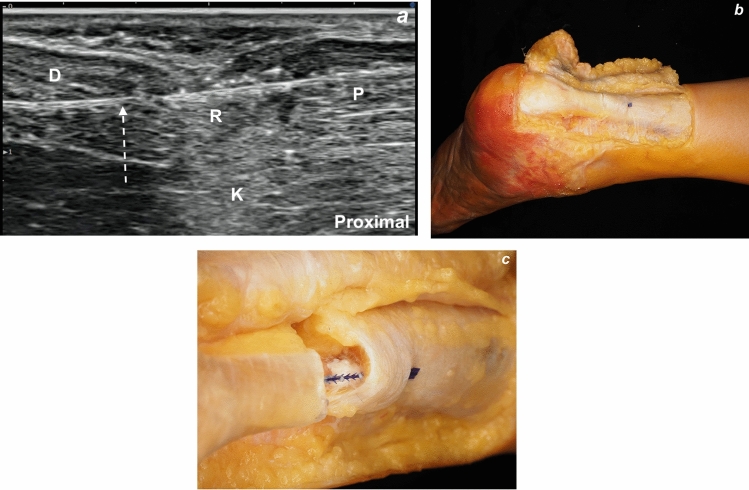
a, b, c Confirmation of tendon ends approximation following ultrasound-guided repair. a Ultrasound image of the Achilles tendon in the longitudinal axis, obtained prior to dissection, showing appropriate approximation of the tendon ends without residual gapping. b Cadaveric image of the dissected Achilles tendon in a resting position, showing that the tendon ends were in direct contact, confirming successful reapproximation. c Intratendinous dissection showing that the suture was accurately positioned in the middle of the tendon substance. P: proximal end of the tendon, D: distal end of the tendon, R: rupture site, K: Kager’s fat pad, dashed arrow: barbed suture
